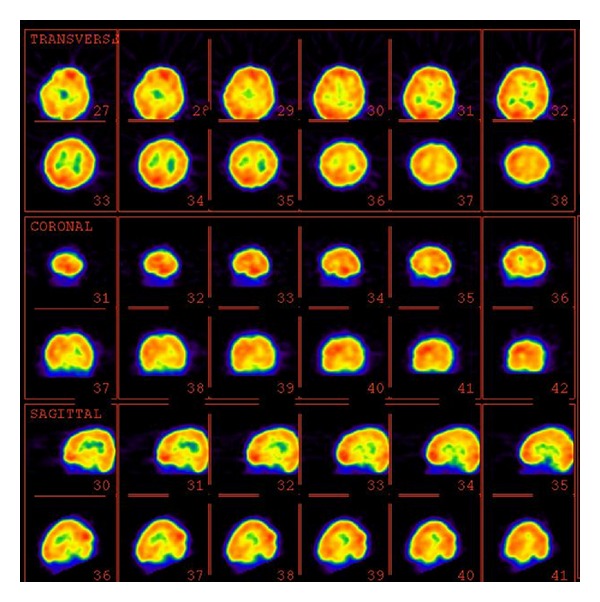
99mTc-ECD brain perfusion SPECT image of a 6-year-old boy with Landau-Kleffner syndrome (LKS). There is moderate hypoperfusion in the left temporal, left parietal, and left inferior frontal regions and also mild hypoperfusion in the right inferior parietal area. The upper rows indicate transverse 99mTc-ECD SPECT images. The middle rows indicate coronal 99mTc-ECD SPECT images. The lower rows indicate sagittal 99mTc-ECD SPECT images.
